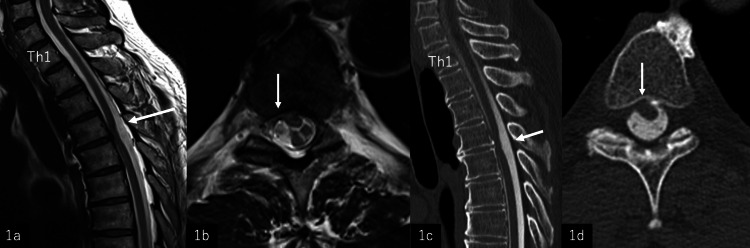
MRI and CT myelogram at initial visit 1a: T2-weighted midsagittal MRI at initial visit. Anterior deviation of the spinal cord is observed from the T4 to T5 level (arrow). 1b: T2-weighted axial MRI at the T4-T5 level at initial visit. The spinal cord is displaced anteriorly, and the anterior subarachnoid space has disappeared (arrow). 1c: Sagittal CT myelogram. The spinal cord is displaced anteriorly at the T4-T5 level and adheres to the arachnoid and dura mater (arrow). 1d: Axial CT myelogram at the T4-T5 level. The spinal cord is displaced right-anteriorly and adheres to the arachnoid and dura mater on the right side (arrow).
